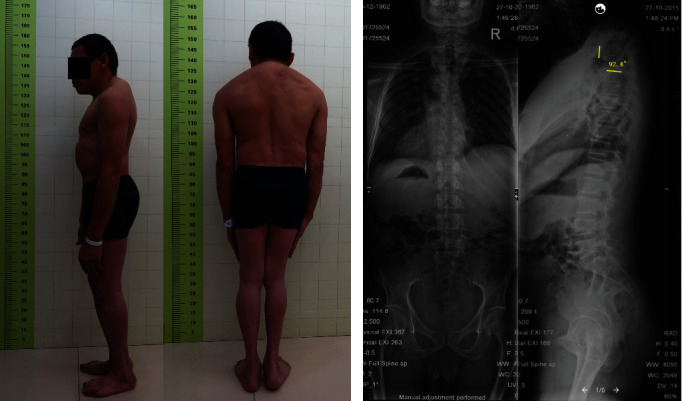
A 60-year-old male patient suffered from rapid deterioration of neurological symptoms with AIS grade C. (a) Clinical images. (b) Plain radiographs showing angular kyphosis of the upper thoracic region of the spine.
